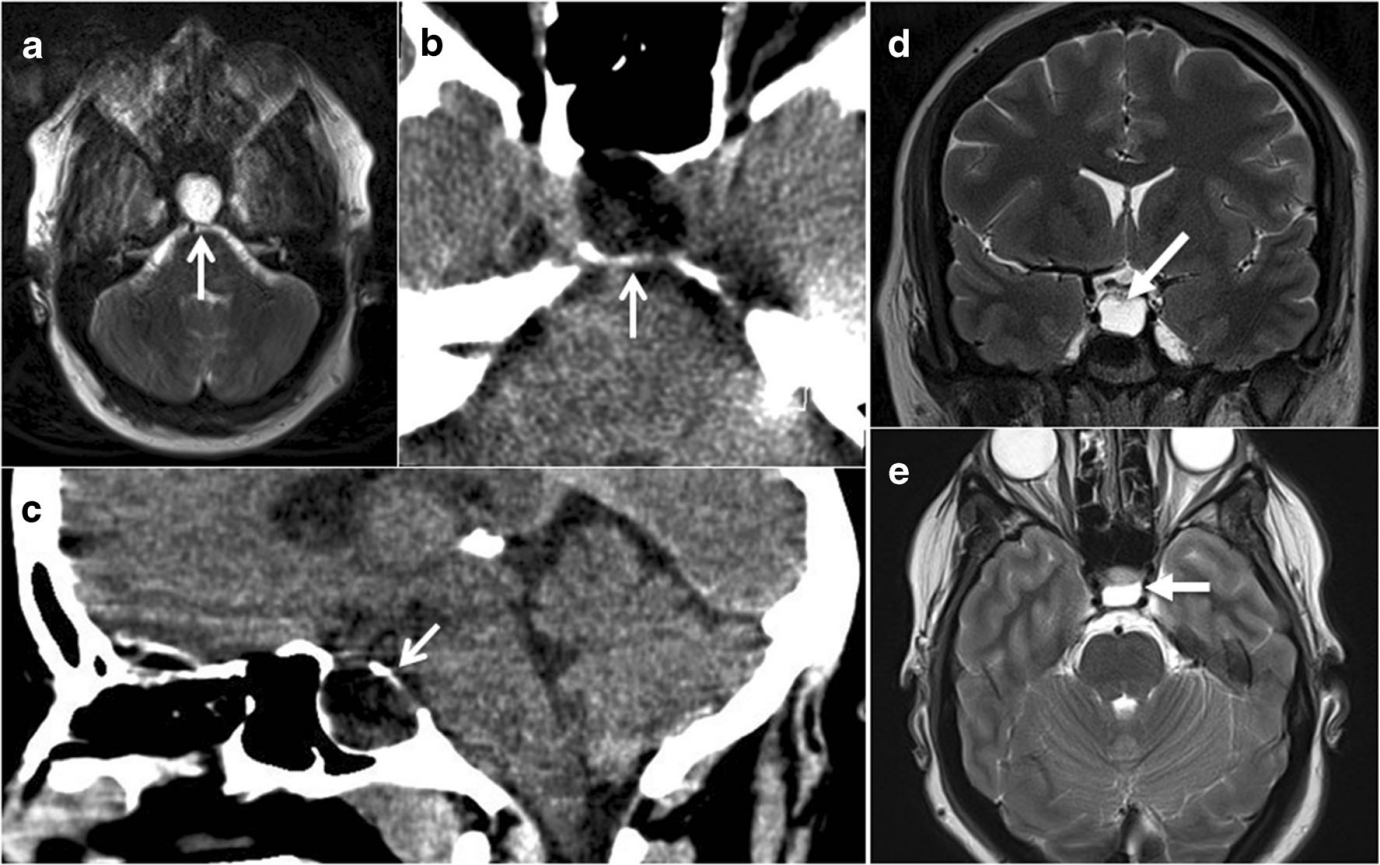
MR imaging. Imaging of proband showing cystic pituitary mass (*open arrow*) a T2-weighted axial MRI, b axial CT, c sagittal CT; imaging of proband’s daughter showing cystic pituitary mass (*solid arrow*) d T2-weighted coronal MRI, e T2-weighted axial MRI
